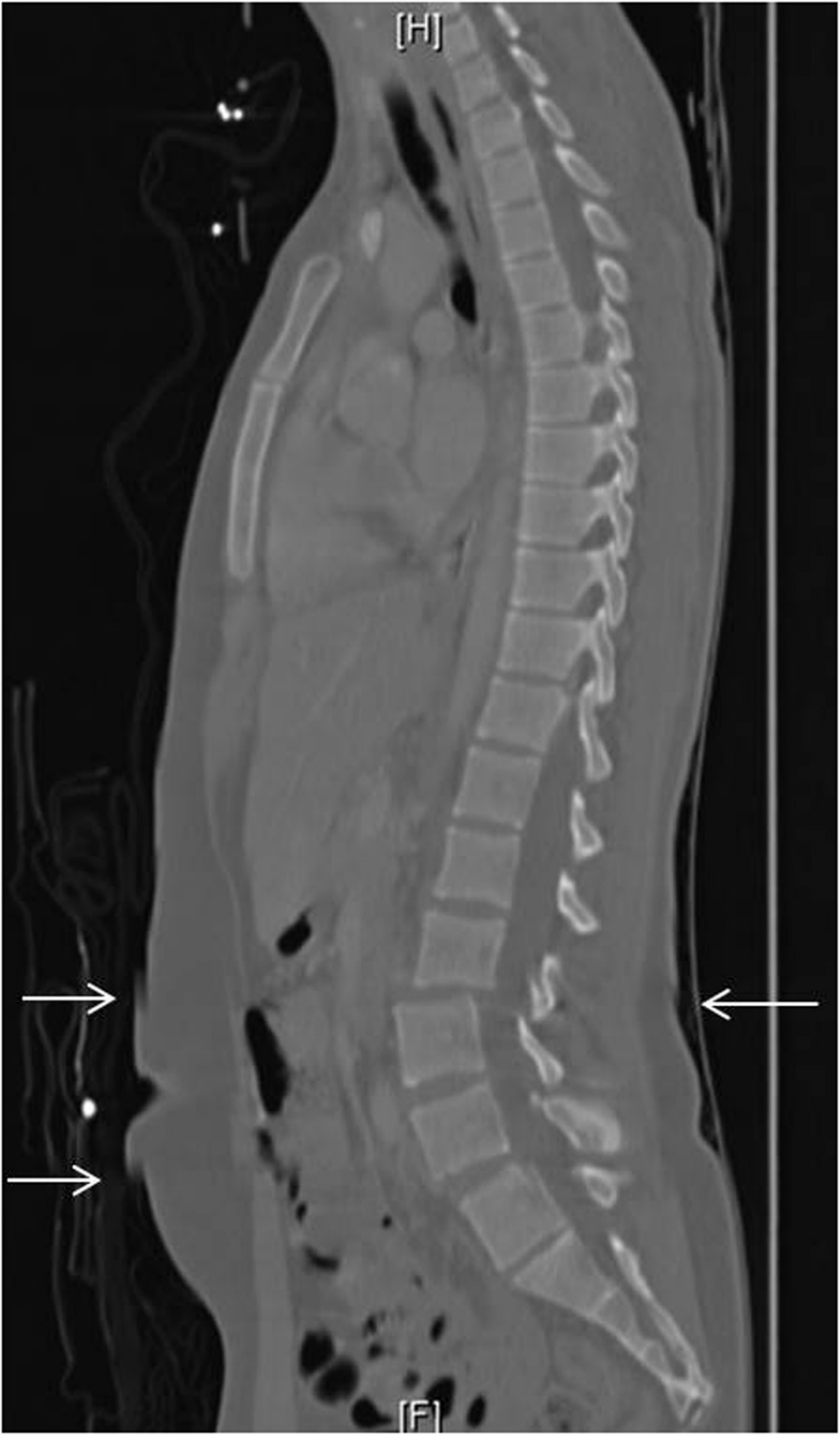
Sagittal reconstruction of abdominal CT scan showing what appears to be a three-column ligamentous injury at L2-3. However, motion artifact can be seen anteriorly and posteriorly (arrows).
